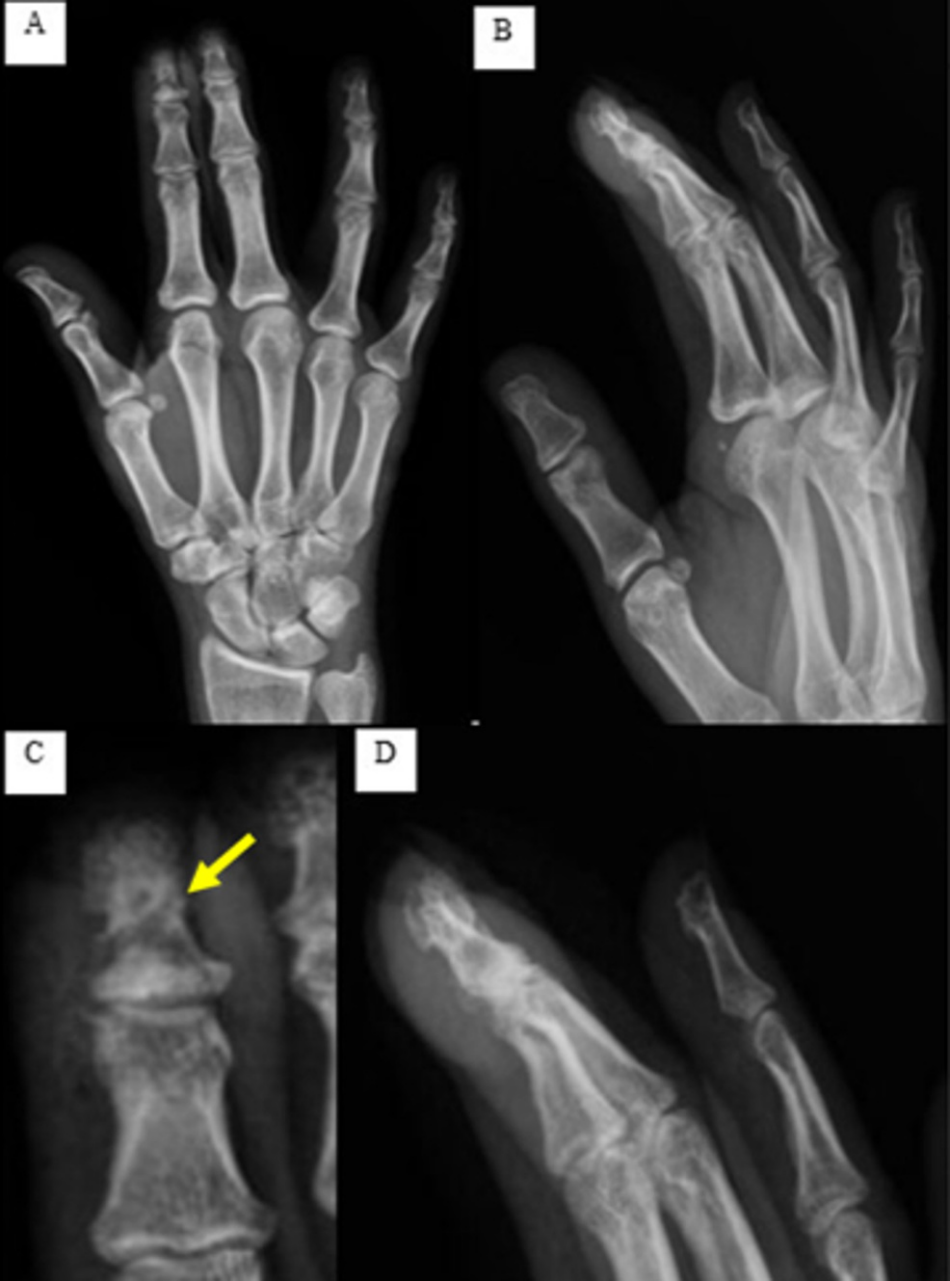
Preoperative radiographs of the right hand. (A) AP view; (B) lateral view; (C) and (D) AP and lateral magnified images, respectively, focusing on the distal phalanx of the index finger showing a lytic lesion of the distal phalanx with surrounding sclerosis (yellow arrow). AP, anteroposterior.
